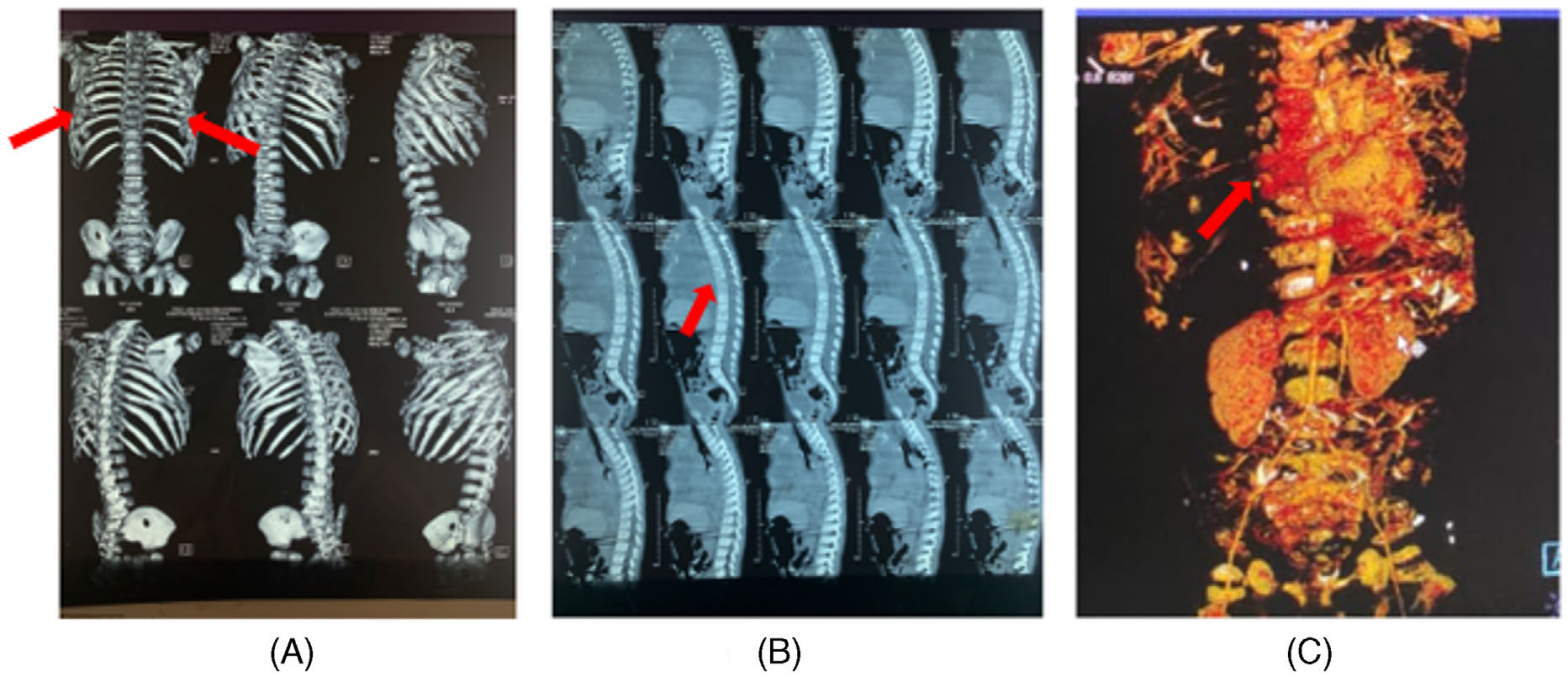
(A) Chest 3D CT‐angiography revealed multiple rib destructions of rib 9, 8, 5, 4, 3, 2 and 1 anterior dextra and rib 4, 3, 2 and 1 anterior sinistra, (B) multiple osteopenia of thoracic vertebrae and (C) vascular mass in the sternum region.
